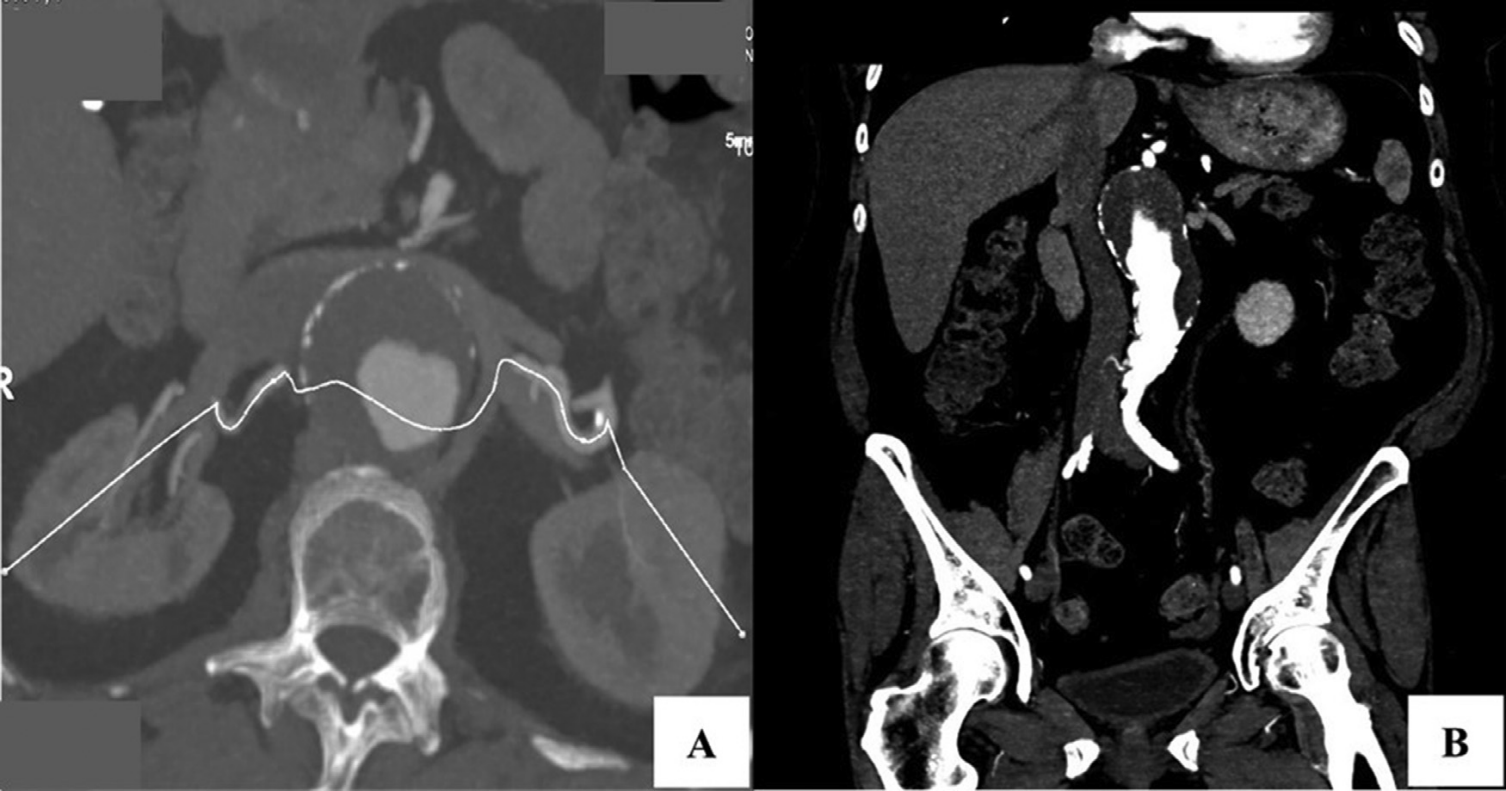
Axial (A) and coronal (B) views of preoperative CTA demonstrating a 5.6 cm Type IV TAA.
